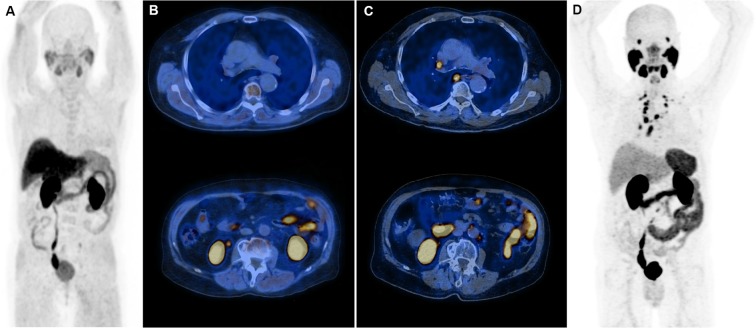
(A) 77-year-old man initially treated by prostatectomy 23 years before PSMA-11 PET/CT (ISUP 1, intermediate risk according to d’Amico classification). The first biochemical recurrence occurred 10 years after the surgery and was treated by radiation therapy of the prostatic lodge, which had no effect on PSA serum level, and androgen deprivation therapy (ADT) by LHRH agonist was initiated (PSA nadir undetectable). (A) rise in PSA appeared 1 year before PSMA-11 PET/CT. ^18^F-flurocholine PET/CT, which was performed 4 months before PSMA-11 PET/CT, was negative (PSA at 4.6 ng/ml) (A: maximum intensity projection view (MIP); (B): axial fusion slices centered on the thorax and the abdomen). PSMA-11 PET/CT (PSA at 5.5 ng/ml) demonstrated multiple malignant foci in the paraaortic region and in lymph nodes located above the diaphragm (D: MIP; C: axial fusion slices centered on the thorax and the abdomen), probably related to prostate cancer. A second generation ADT was started (PSA at 10 ng/ml) and was effective on PSA (at 4.8 ng/ml and stable 15 months after ADT modification).
